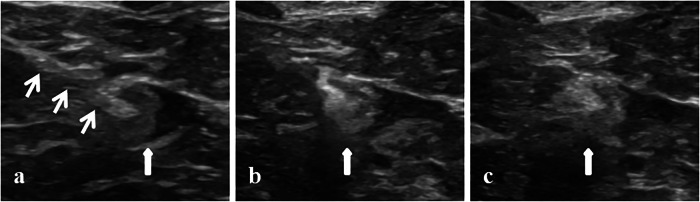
Sonographic monitoring of SLN changes following MB injection. The thin white arrows represent the puncture needle, the thick white arrow represents the SLN. a During puncture. b During injection. c After injection. SLN, sentinel lymph node; MB, methylene blue
